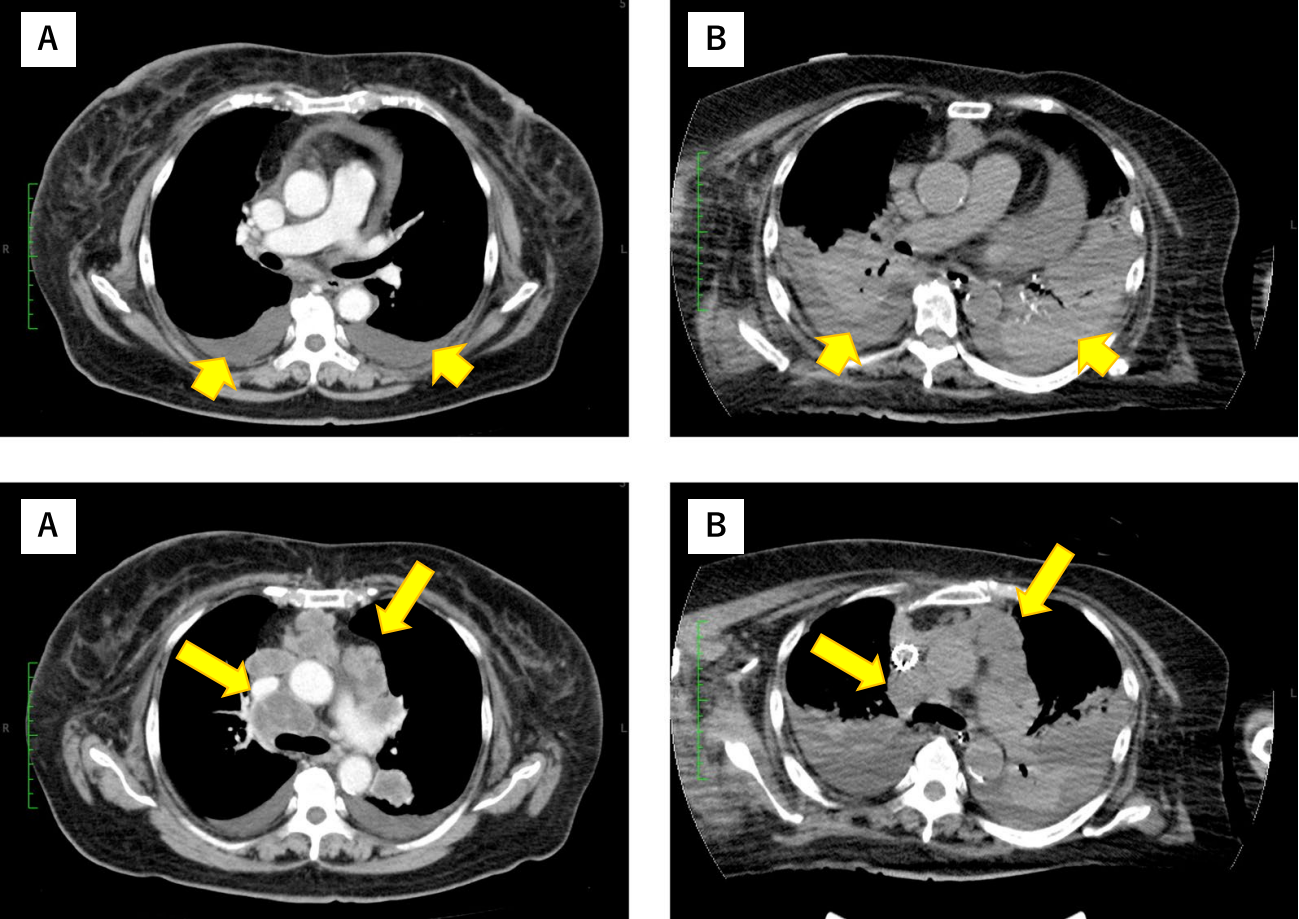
Chest computed tomography images before and after administering tepotinib. a Primary lung cancer in the left lower lobe, mediastinal lymph node metastasis, and bilateral pleural effusions before treatment. b Tumor progression in mediastinal lymph node metastasis (long arrow) and bilateral pleural effusion (short arrow)
